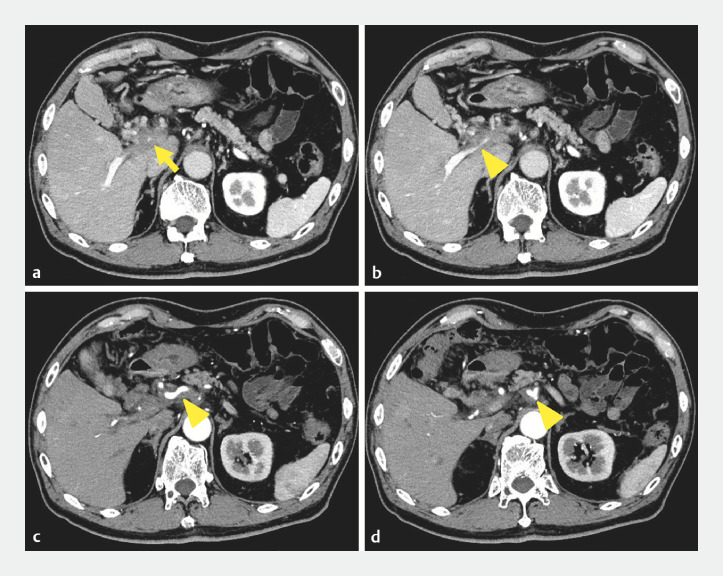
Contrast-enhanced computed tomography images (
